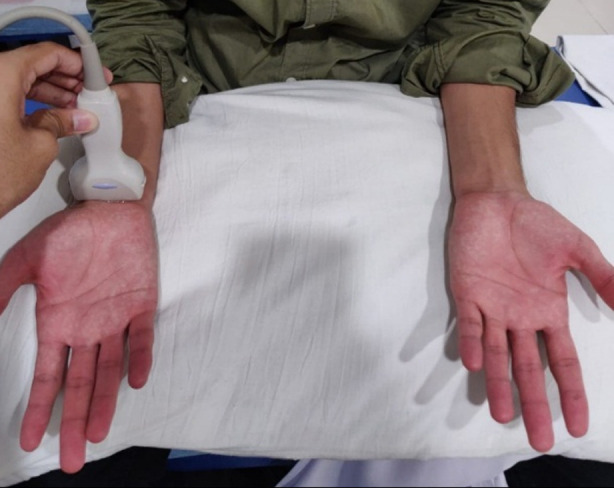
A subject undergoing ultrasound of right wrist at the distal wrist crease level (carpal tunnel inlet).
